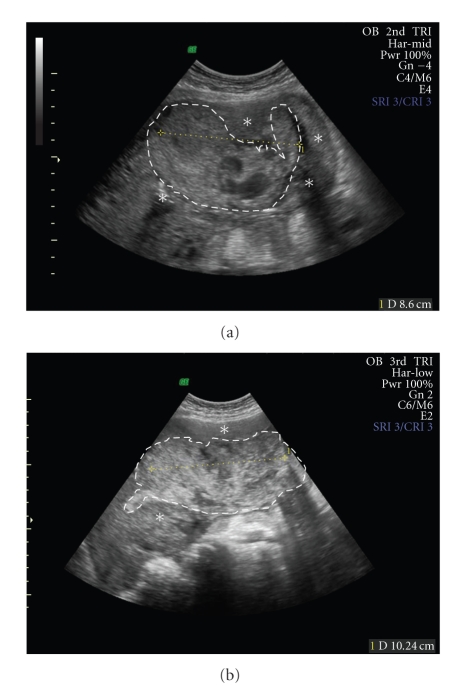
Transabdominal ultrasound image taken on postpartum day 17. This view of the uterine fundus demonstrates significant persistence of retained placental tissue approximately two weeks after methotrexate administration. Serum *β*hCG was 1571 UI/L. The distinction between the placental mass (dotted line) and myometrium (asterisk) is shown. Transabdominal ultrasound image taken on postpartum day 24. This view of the uterine fundus shows significant persistence of retained placental tissue approximately three weeks after methotrexate administration. Serum *β*hCG was 479 UI/L. The distinction between the placental mass (dotted line) and myometrium (asterisk) is depicted.
